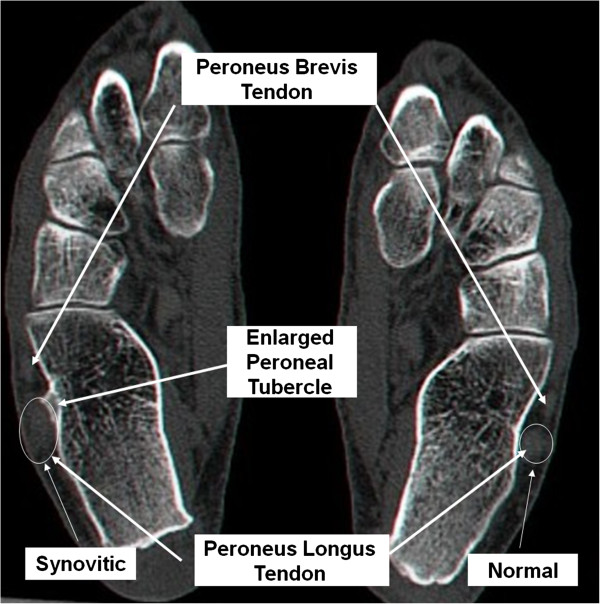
Axial CT scan view showing the enlarged peroneal tubercle and thickened peroneus longus.
